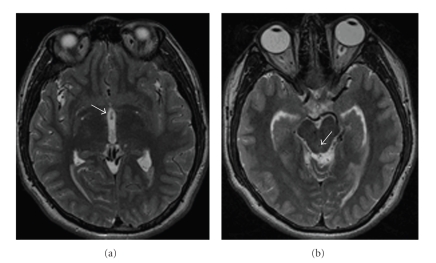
(a) MRI T2 showing migration of the cyst to the anterior third ventricle (arrow) and (b) MRI T2 demonstrating a flow void in the Sylvian aqueduct consistent with restoration of CSF flow (arrow). Of note, reduction in the size of the temporal horns is also appreciated.
